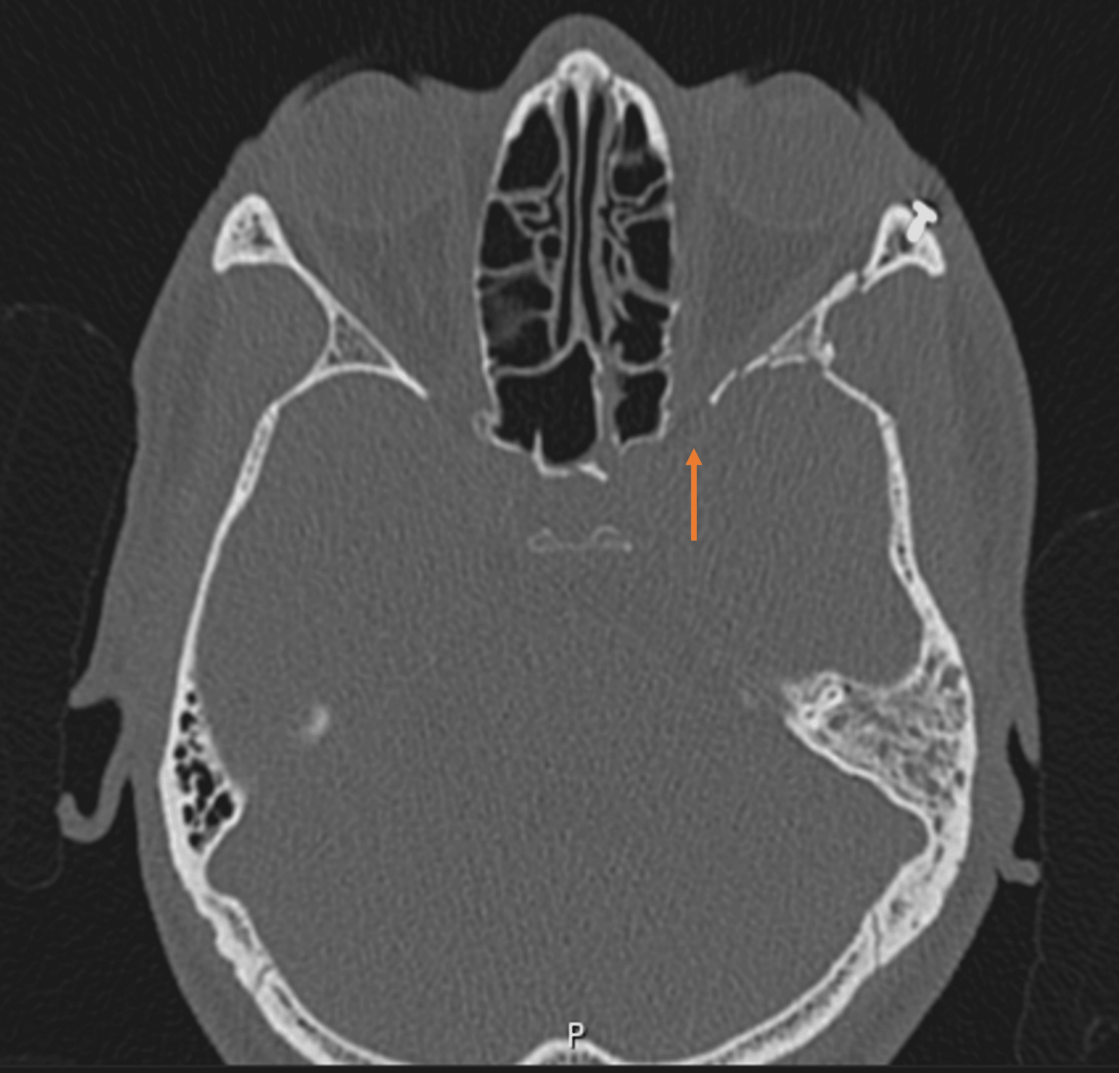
Postoperative CT imaging (axial view). The orange arrow highlights the postoperative normalization of the superior orbital fissure's traumatic narrowing.
